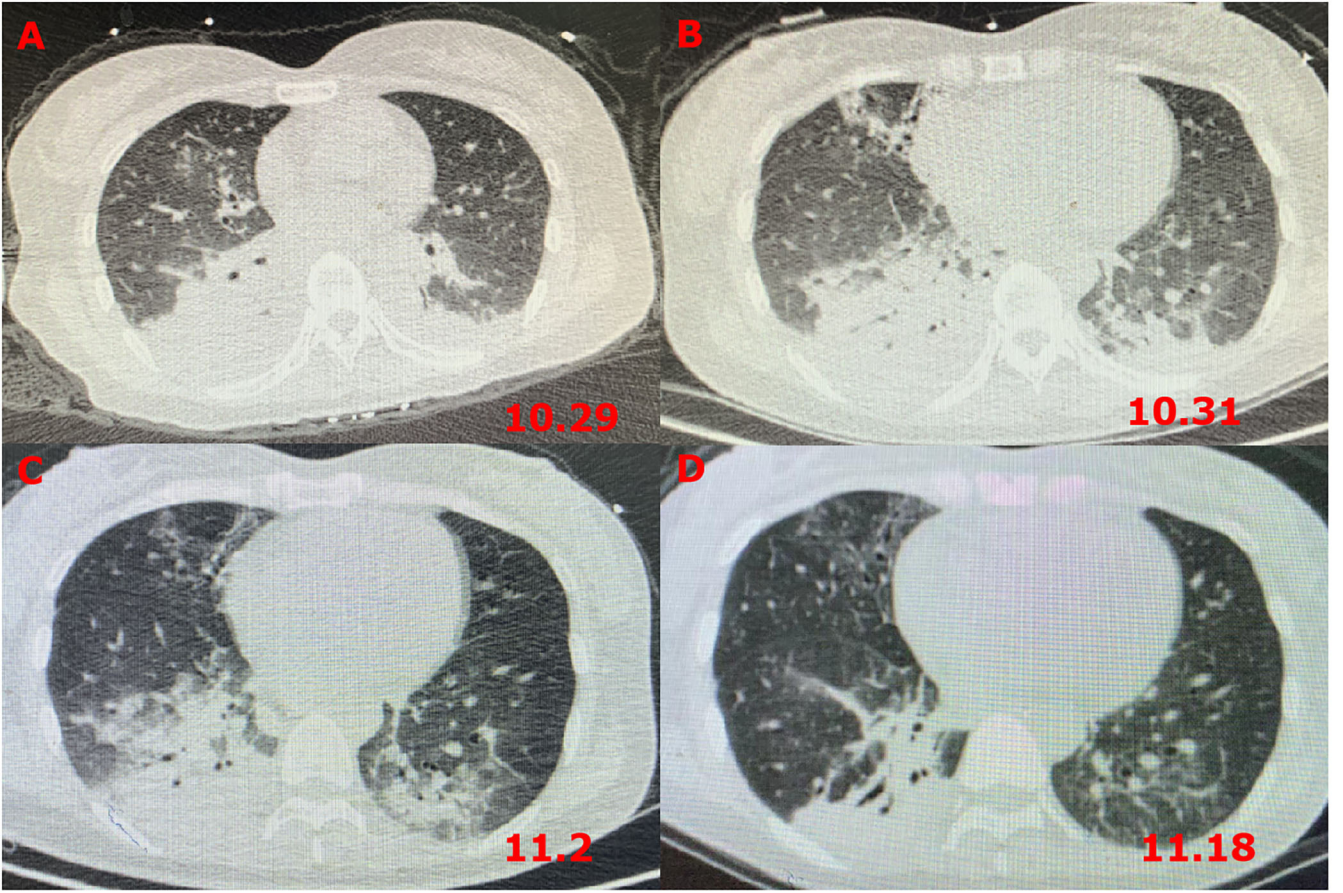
Chest CT images revealing pneumonia. (A) CT scans on the first day after admission; (B) CT scans at 72 h after admission; (C) CT scans on the fourth day after transferring out of the ICU; and (D) CT scans after GBS diagnosis and treatment for 5 days.
